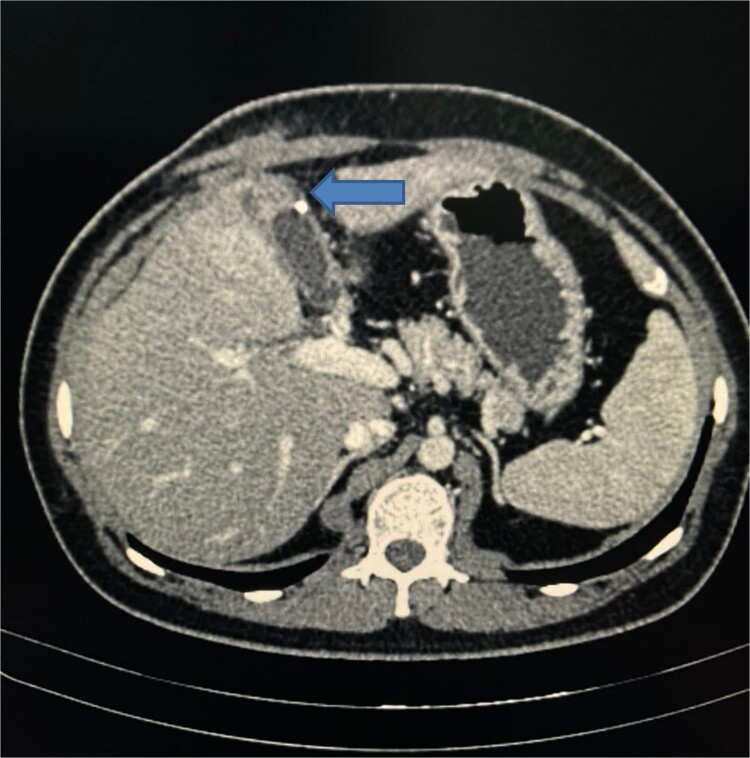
CT scan of abdomen showing a walled-off fluid collection with a small calcific density and a soft tissue density tract-like lesion extended from the anterior aspect of the collection towards the subcutaneous plane of the right upper abdominal wall, suggesting a sinus tract.
